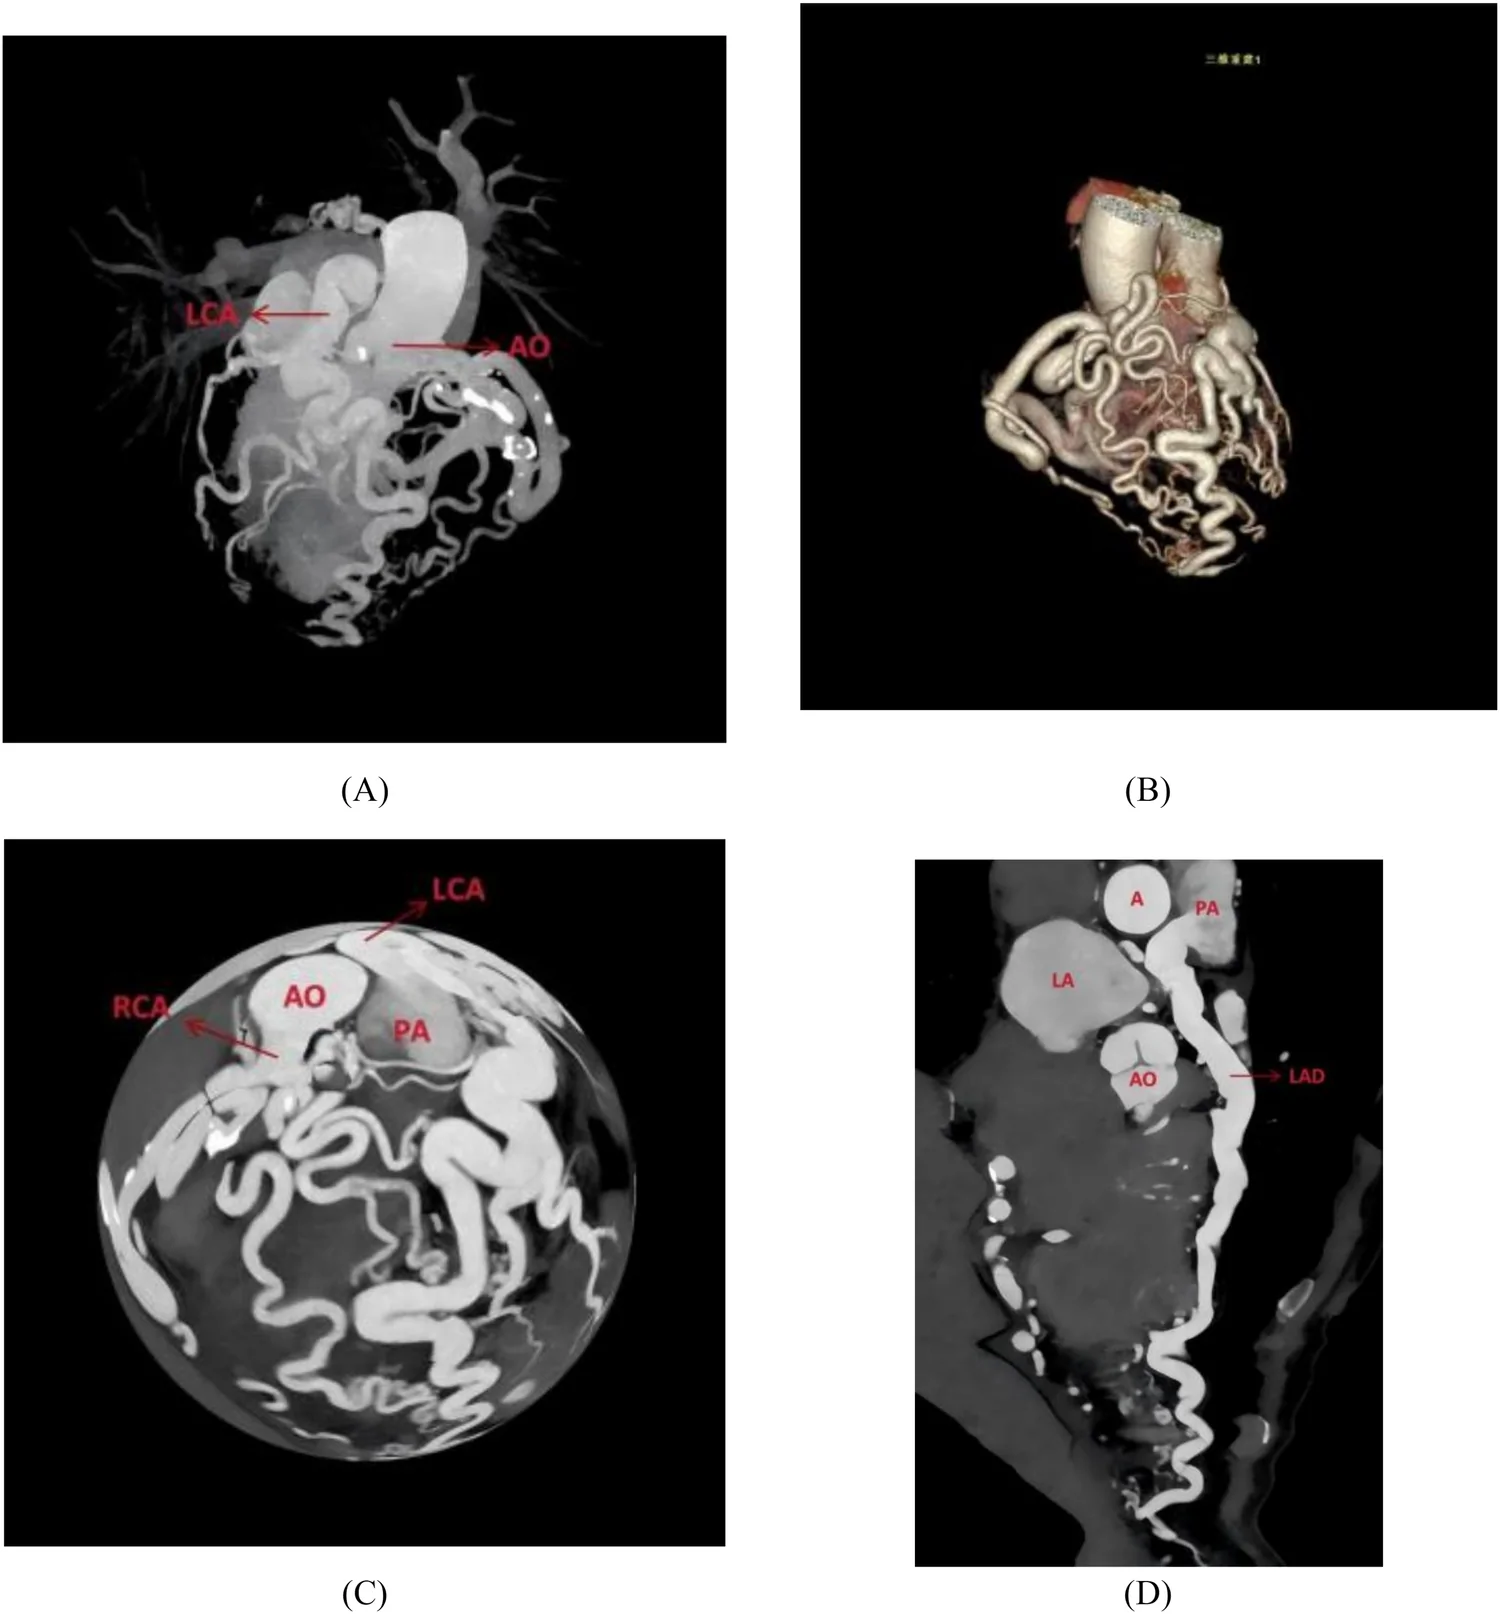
Coronary CTA in a 76-year-old man with ALCAPA. (A) Maximum intensity projection image showing anomalous origin of the left main coronary artery (LMCA, arrow) from the pulmonary artery (PA), providing direct anatomical evidence of ALCAPA. (B) Volume-rendered reconstruction showing a markedly dilated and tortuous right coronary artery (RCA) and extensive epicardial collateral vessels. (C) Panoramic coronary reconstruction showing collateral filling of the left coronary system, including the left anterior descending and left circumflex arteries. (D) Curved planar reformation of the LMCA confirming its origin from the pulmonary artery and delineating the proximal anomalous vessel. The figure demonstrates anatomical collateralization but does not by itself establish absence of inducible ischemia, myocardial scar, or coronary steal physiology.
